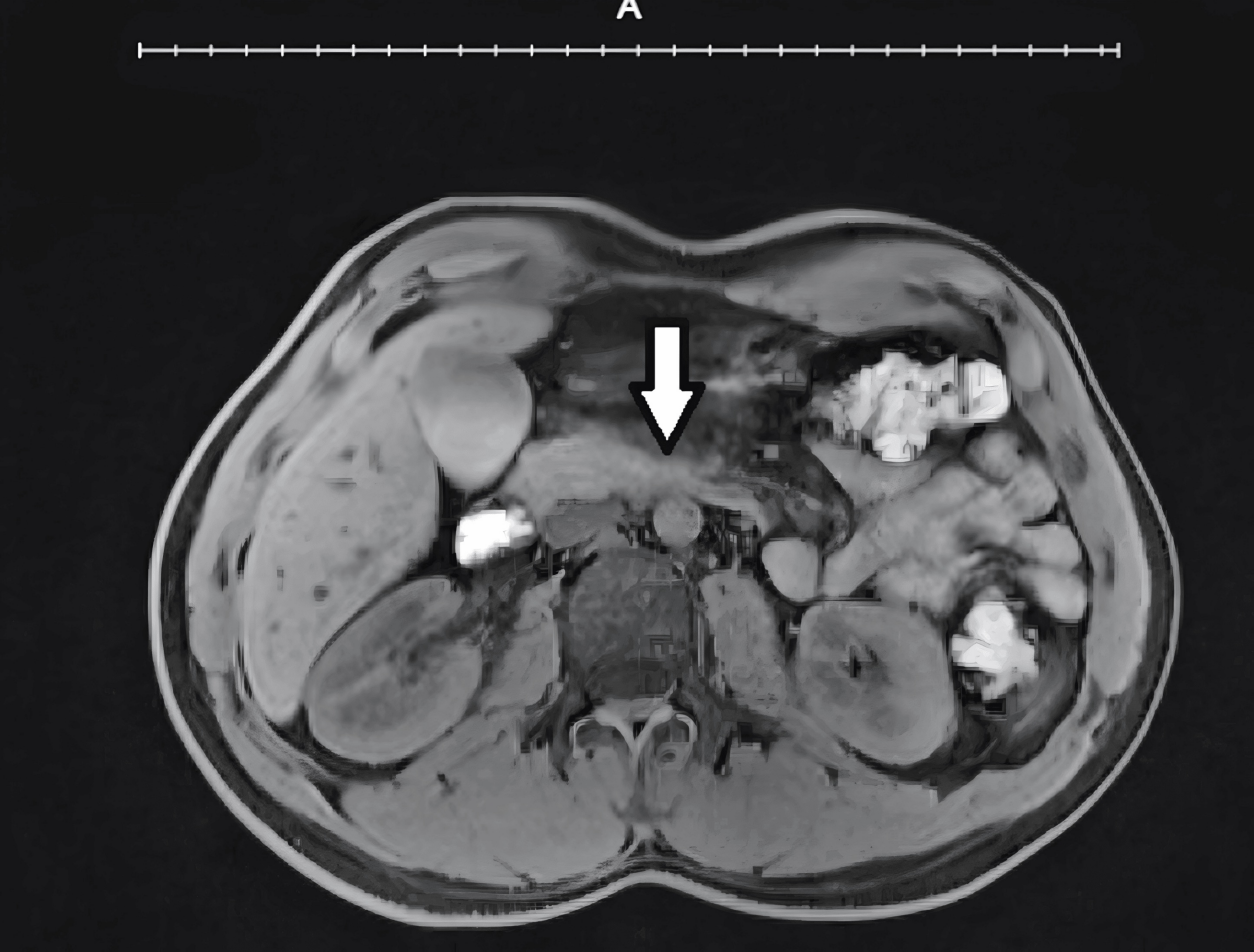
Axial T2-weighted MRI reveals increased signal intensity in the pancreas, indicating inflammatory edema. Hyperintense peripancreatic fluid collections are noted, along with hyperintense inflammatory stranding within the peripancreatic fat, consistent with acute pancreatitis. The arrow indicates the inflamed pancreas.
